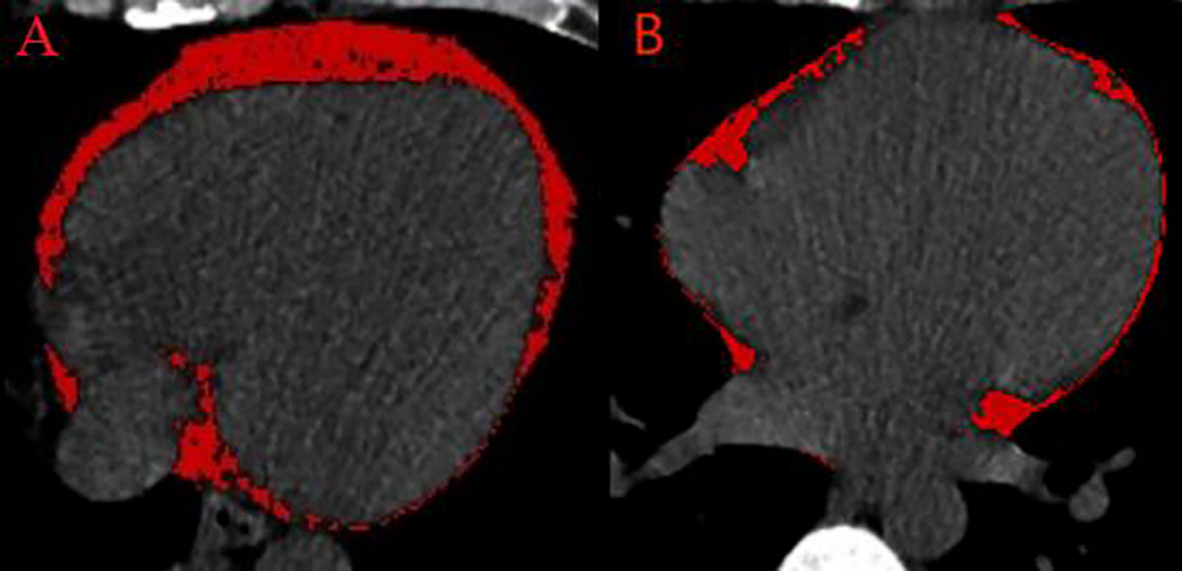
Semiautomated EAT volume analysis on non-contrast CT. (A) A patient with frequent PVCs. (B) A control patient. CT, computed tomography; EAT, epicardial adipose tissue; PVCs, premature ventricular complexes.
